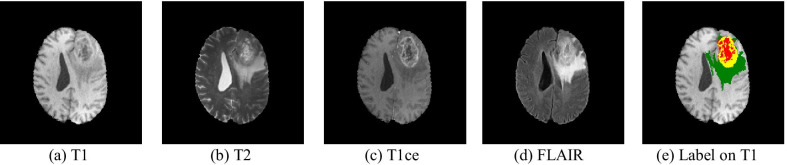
Visualization of one patient in four modalities in BraTS2020 training Dataset. (a) T1 MRI, (b) T2 MRI, (c) T1ce, (d) FLAIR MRI, and the label shown in T1 MRI
